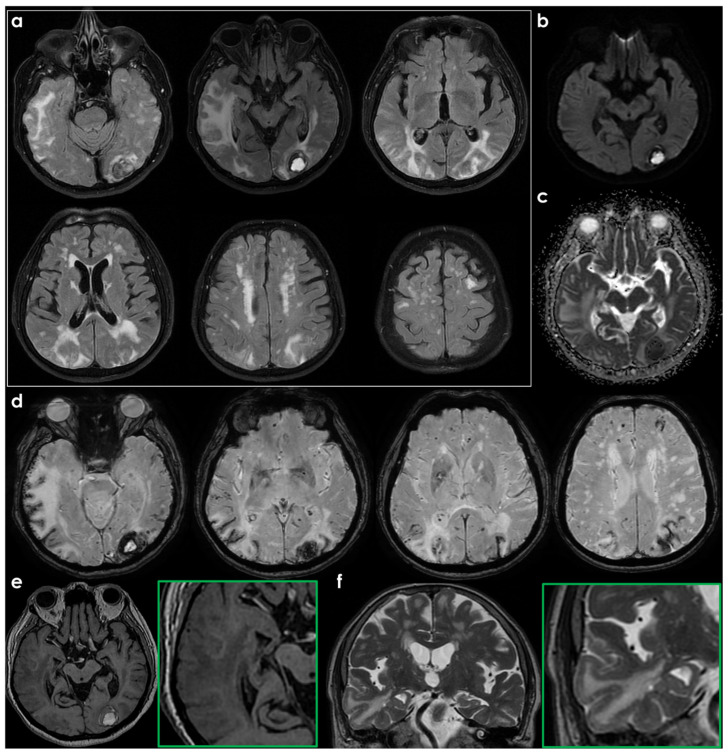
Brain MRI of a patient (male, 77 years old, past history of arterial hypertension and smoking habitus) referred to the neurologist because of a slowly progressive global impairment of the cognitive performance lasting three months. In panel (a), the axial FLAIR slices in a progressively ascending direction show multilobar, bilateral and mildly asymmetric white matter hyperintensities in the subcortical location, with tumefactive effect, superimposed on a pattern of chronic WMHs of presumed vascular origin in the centrum semiovale on both sides and deep lacunae. In the left occipital pole, within the above described white matter tumefactive lesions, a subacute ICH is visible as rounded hyperintese focal lesion. In panel (b,c), respectively, DWI and ADC images are proposed, highlighting the signal features supportive of vasogenic edema, corresponding to tumefactive white matter hyperintensities in the temporal lobe on both sides. In panel (d), SWI shows diffuse cSS, mainly corresponding with the ARIA-E topography, and in the regions the left occipital ICH. In panel (e,f), axial T1WI and coronal T2WI and magnified details, show the extension of the edema to the cortico-subcortical junction.
